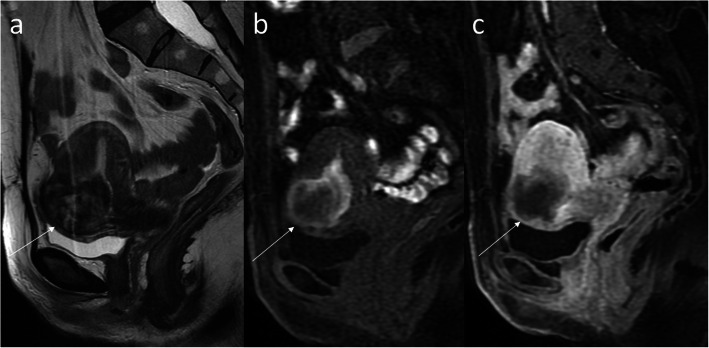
Pelvic MRI in the sagittal plane, FSE/T2WI (a). Dynamic contrast-enhanced fat-saturated T1 sequence before (b) and after (c) gadolinium administration. Retained products of conception (RPOC) of mixed signal intensity in the C-section niche after treatment with potassium chloride and methotrexate (arrows)
